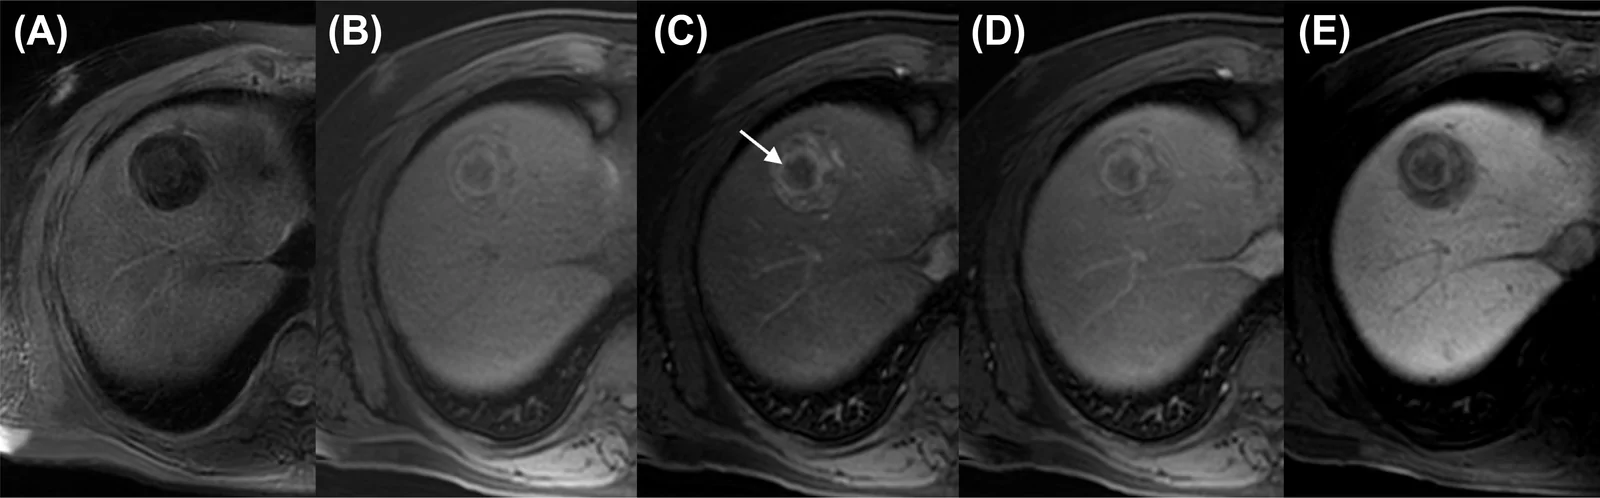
In gadoxetic acid-enhanced MRI of the liver, (A) the T2-weighted image shows heterogeneous low signal intensity of the lesion, while (B) the T1-weighted image reveals heterogeneous high signal intensity. (C) In the arterial phase, the lesion demonstrates strong enhancement with a nodule-in-nodule appearance (arrow), followed by (D) washout appearance on the portal venous phase. (E) The hepatobiliary phase image shows hypointensity of the lesion.
